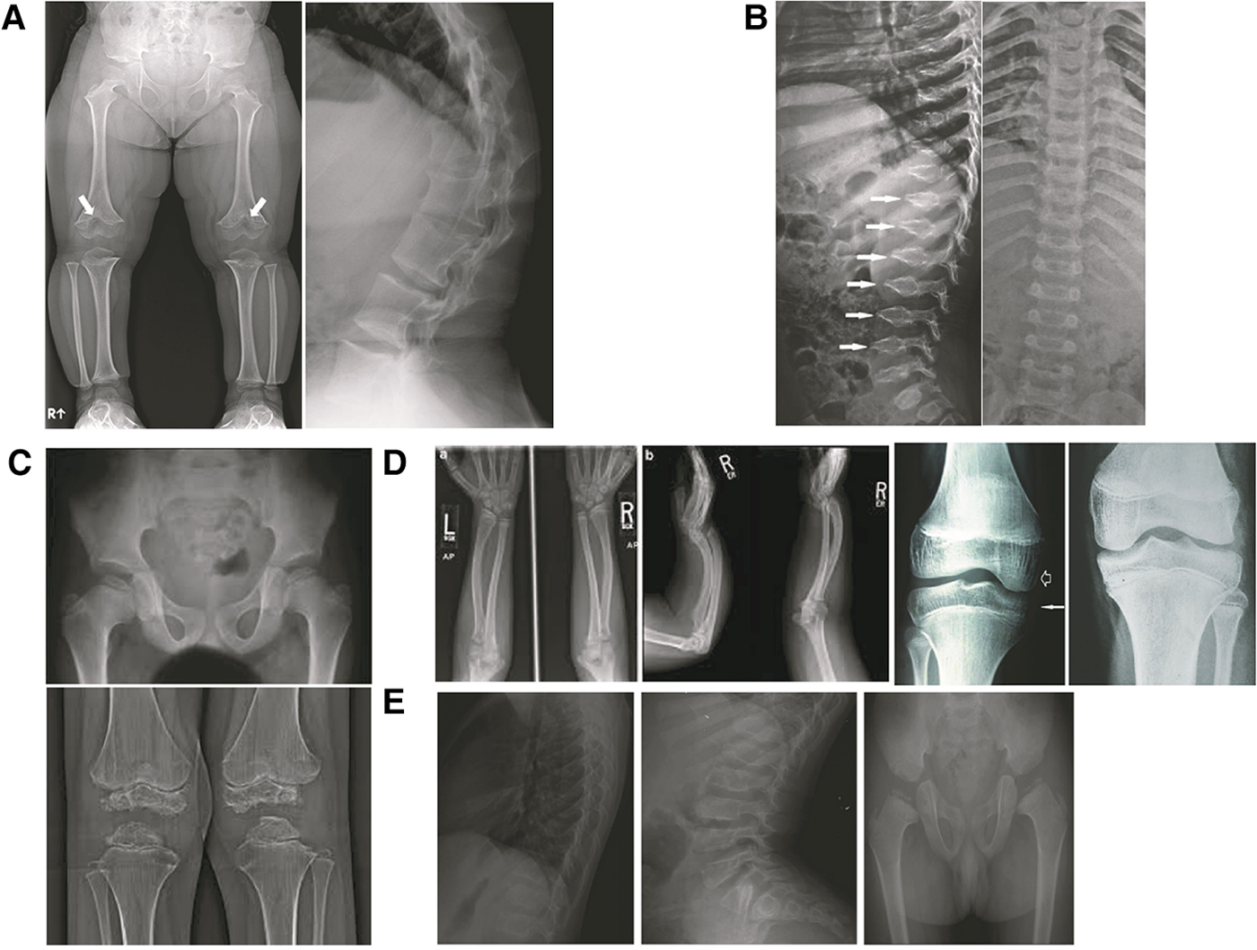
X-ray findings in various skeletal dysplasias: (A) achondroplasia: note chevron shape of distal femur physes, flat acetabular roof, relatively narrow sacroiliac notch, broad pelvis, metaphyseal flaring, short robust bones, short pedicles on lateral spine film; (B) pseudoachondroplasia: note the platyspondyly and oval vertebral bodies with anterior beaking (C) MED: bilateral femoral heads, distal femoral epiphyses and proximal tibial epiphyses small and ragged; (D) SHOX: madelung deformity, downward displacement of distal femur medial condyles; (E) SEDC: flattened vertebrae (platyspondyly), elongated vertebral bodies, late appearing and small femoral heads.
